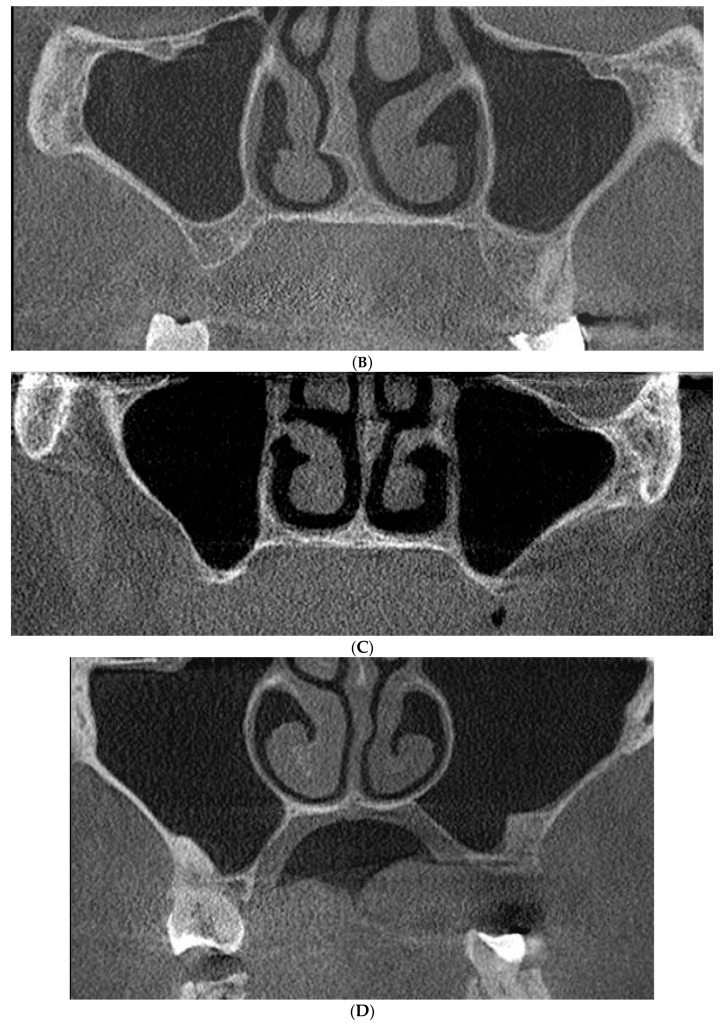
Evaluation of the maxillary sinus based on cone beam computed tomography (CBCT) scans in the coronal view. (A) Class 0: Adequate sub-sinus alveolar bone height. (B) Class 1: Reduced sub-sinus alveolar bone height with a thin lateral wall. (C) Class 2: Reduced sub-sinus alveolar bone height with comparable thickness of the lateral and palatal walls. (D) Class 3: Reduced sub-sinus alveolar bone height with a thin palatal wall.
